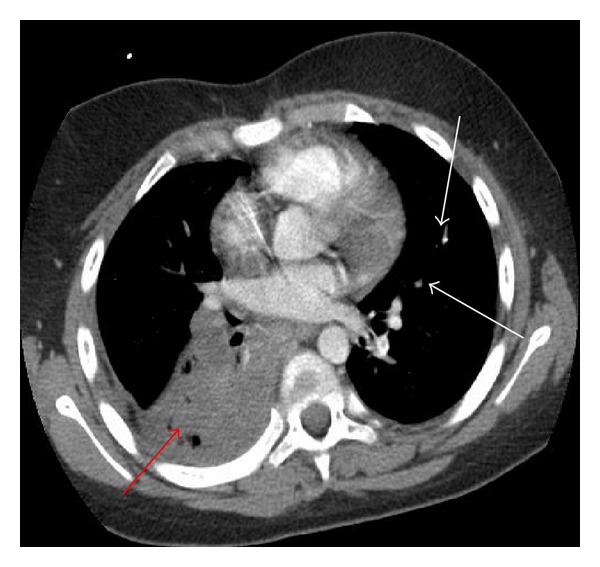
CT Chest with pulmonary angiogram. There is complete occlusion of the descending branch of the right pulmonary arteries to the right lower lobe (Red arrow). Multiple small pulmonary artery emboli were also seen in the left lobe (White arrows).
